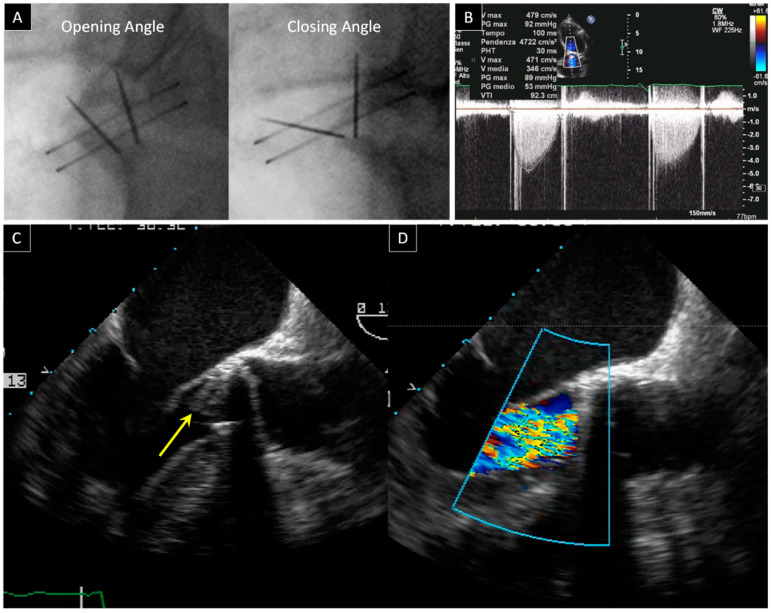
Bileaflet mechanical aortic valve obstruction. (A) Fluoroscopy shows normal opening and abnormal closing angles in a patient with prosthetic aortic valve dysfunction. (B) Flow acceleration of the anterograde flow is identified with color flow imaging from the TTE apical approach and it is associated with high transprosthetic gradients at continuous wave Doppler (ΔP_mean_ 53 mmHg). (C) 2D TEE from a 120° view reveals a thrombotic hyperechogenic mass on the ventricular side of the prosthesis (arrow). (D) Severe intraprosthetic regurgitation as assessed by 2D TEE.
